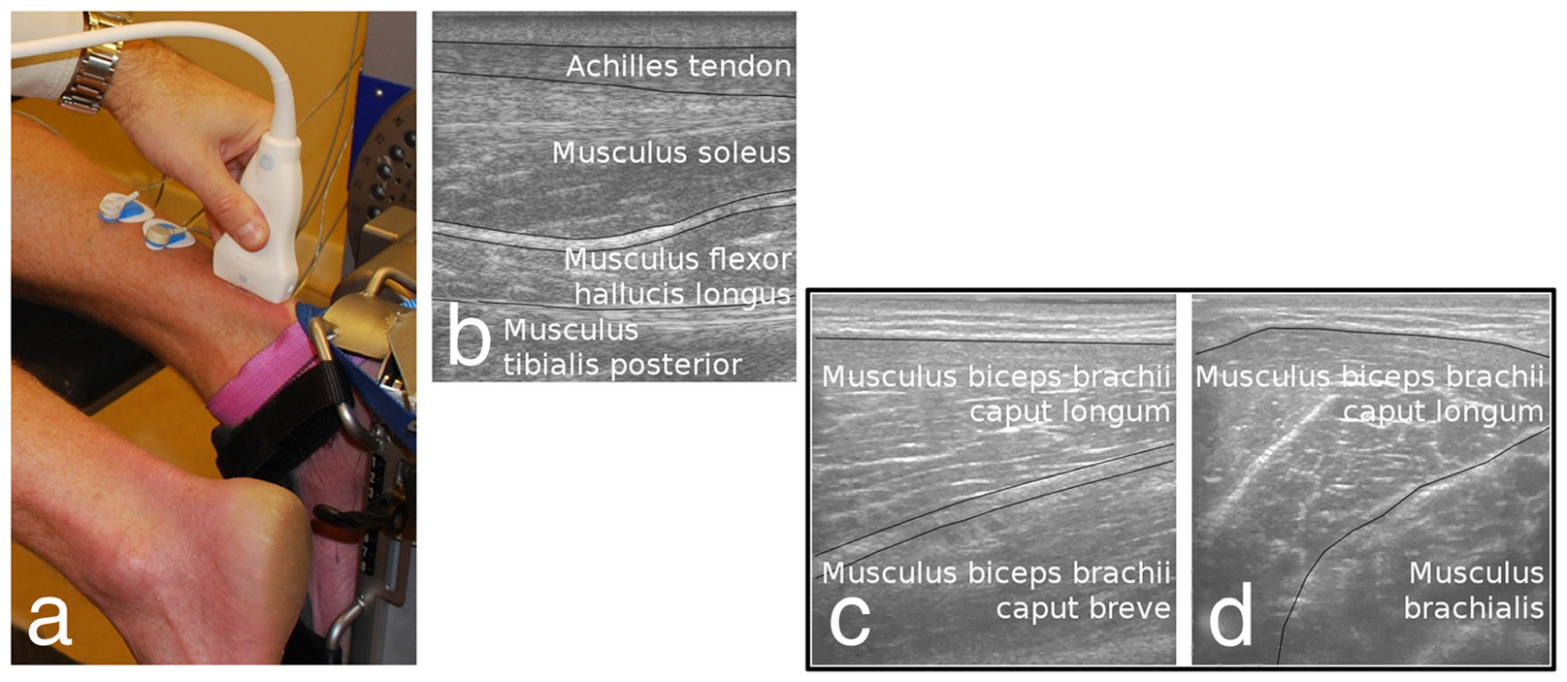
Experimental setup and the resulting ultrasound sequences. (a) The passive movement (Example 1) was performed lying face down with the foot strapped to an isokinetic dynamometer. The ultrasound probe was then held in the researcher’s hand, just above the subject’s malleolus. (b) The anatomy of the calf viewed from the probe surface towards the interior of the calf. The marked anatomic areas are the areas discussed in Example 1. Figures (c) and (d) show the anatomical regions in the biceps considered in Example 2. (c) is the longitudinal capture and (d) is the transversal capture.
